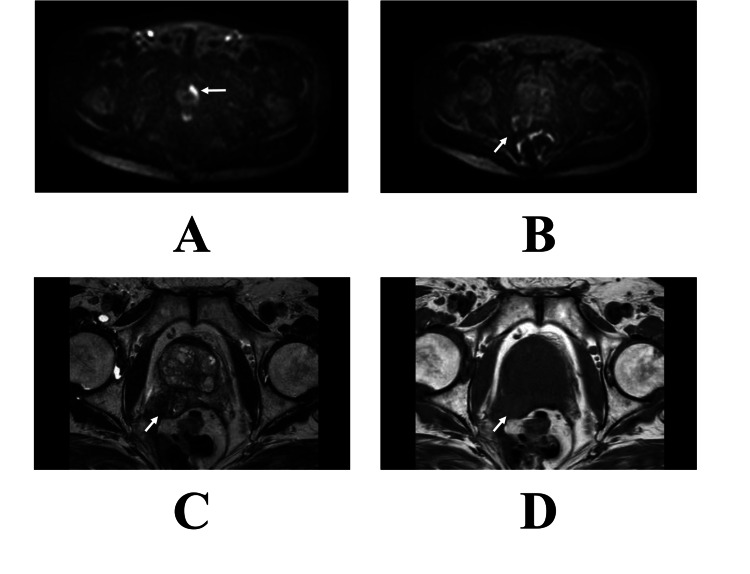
MRI findings A. MRI shows high signals on diffusion-weighted images in the left transition zone (arrow). B. MRI shows predominant low signals on diffusion-weighted images in the right periphery zone (arrow). C. MRI shows a presumed metastatic mass with low signals on T1-weighted coronal images in the right periphery zone (arrow). D. MRI shows low signals on T2-weighted images, presumably corresponding to the metastatic tumor (arrow). MRI, magnetic resonance imaging
